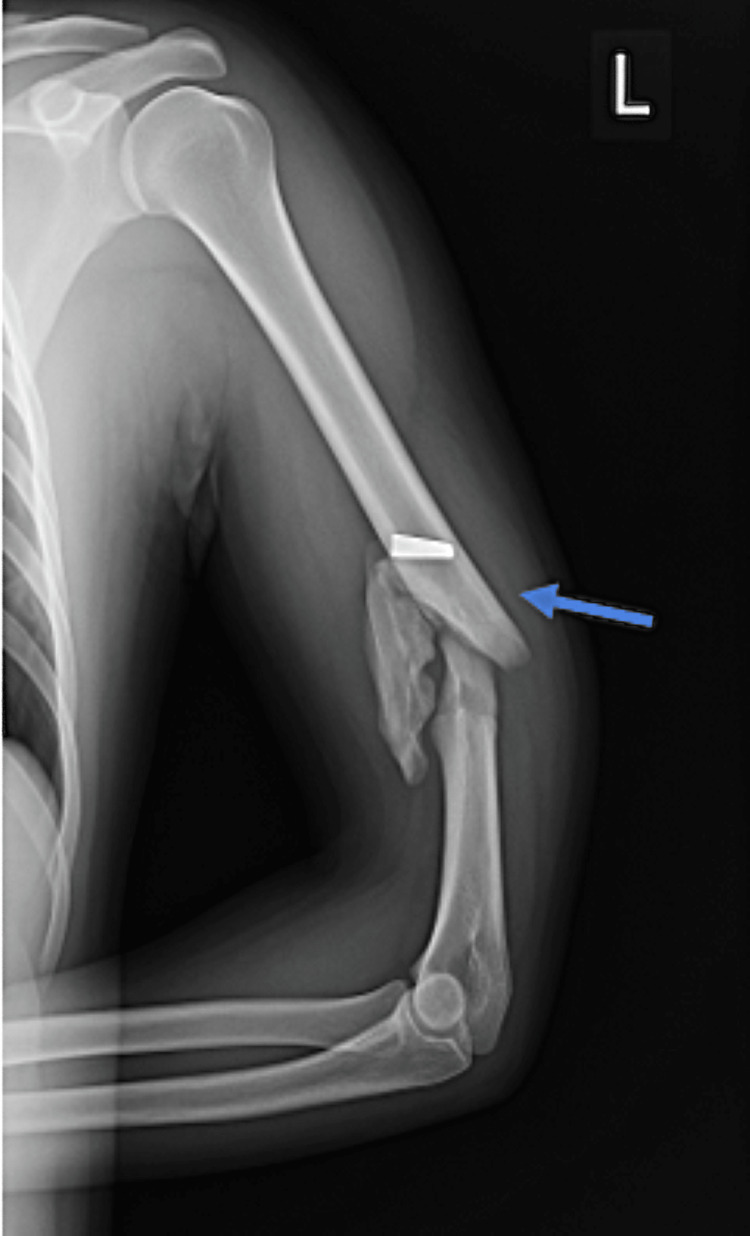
Radiograph of the upper limb demonstrating a comminuted mid-diaphyseal fracture of the humerus (blue arrow) with a retained bullet fragment lodged within the soft tissues of the arm
